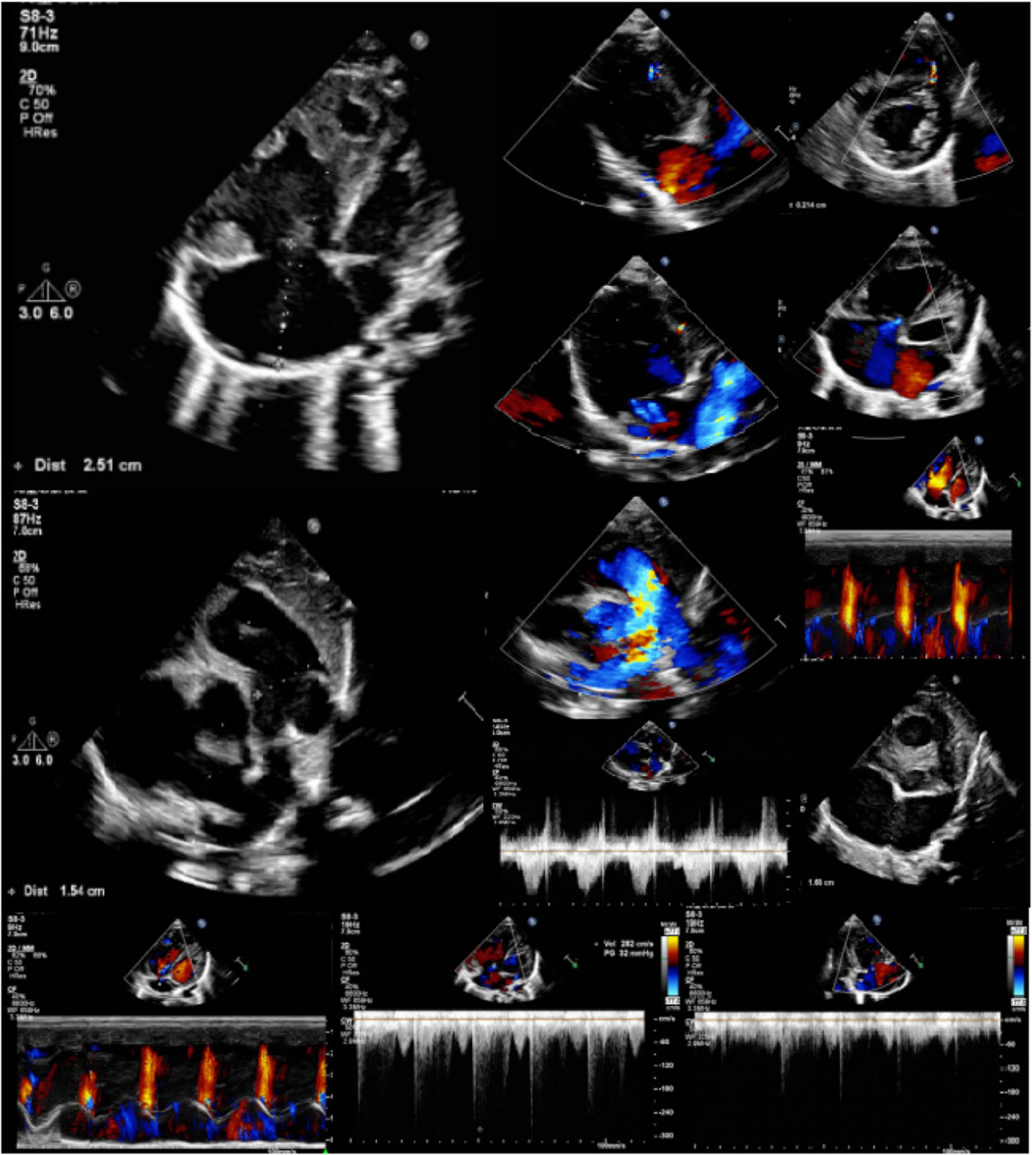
Echocardiography showed atrial septal defect, ventricular septal defect, tricuspid regurgitation (mild to moderate), mitral regurgitation (mild), pulmonary hypertension, biventricular hypertrophy
